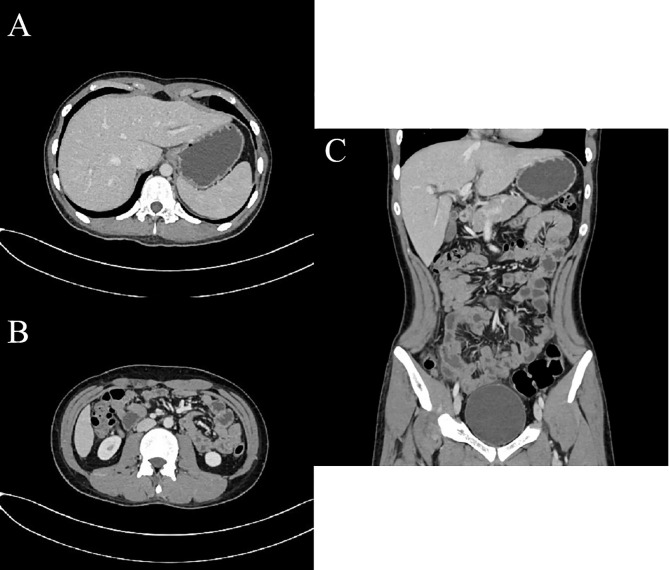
CT examination post-treatment. Axial (A), Axial (B), and coronal (C) enhanced CT images of the abdomen and pelvis in the portal phase post-treatment demonstrated resolution of ascites, thickening of peritoneum and omentum disappeared and turned to the normal shape.
